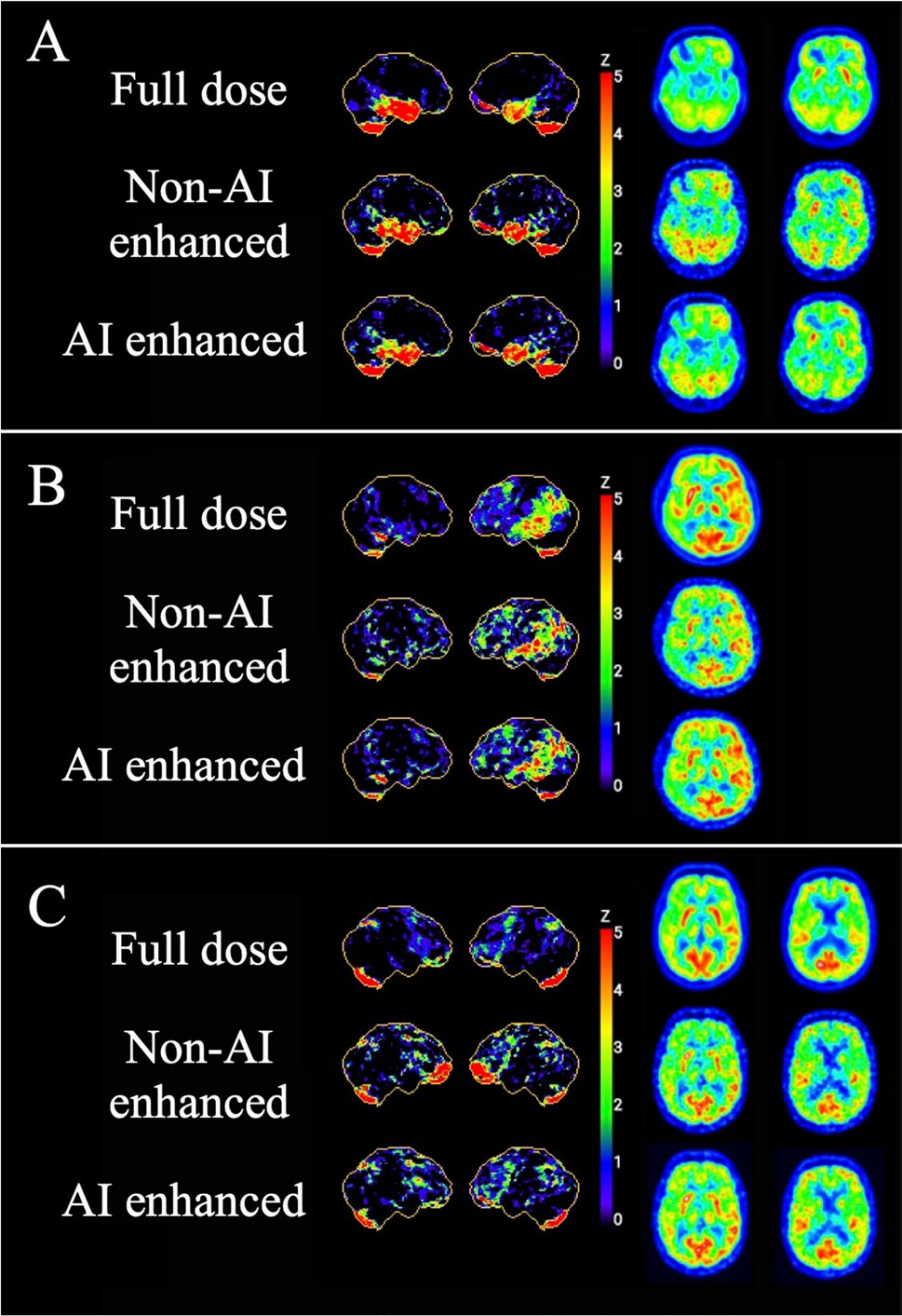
Example [^18^F]FDG images of 3D-SSP results with global cortex as reference region and corresponding axial slides for full-dose, non-AI-enhanced, and AI-enhanced images at DRF = 50 from mCT (Siemens, Biograph mCT). A Male, 59 years old, referred with Alzheimer’s disease and additional clinical signs of a frontal lobe disorder. B Male, 68 years old, referred with progressive word finding and memory difficulties, cerebrospinal fluid with pathological amyloid findings in favor of Alzheimer’s disease. Clinically inconclusive findings (DDx Alzheimer’s dementia, frontotemporal dementia, vascular dementia, Lewy body dementia). C Male, 71 years old, referred with a suspicion of progressive supranuclear palsy
